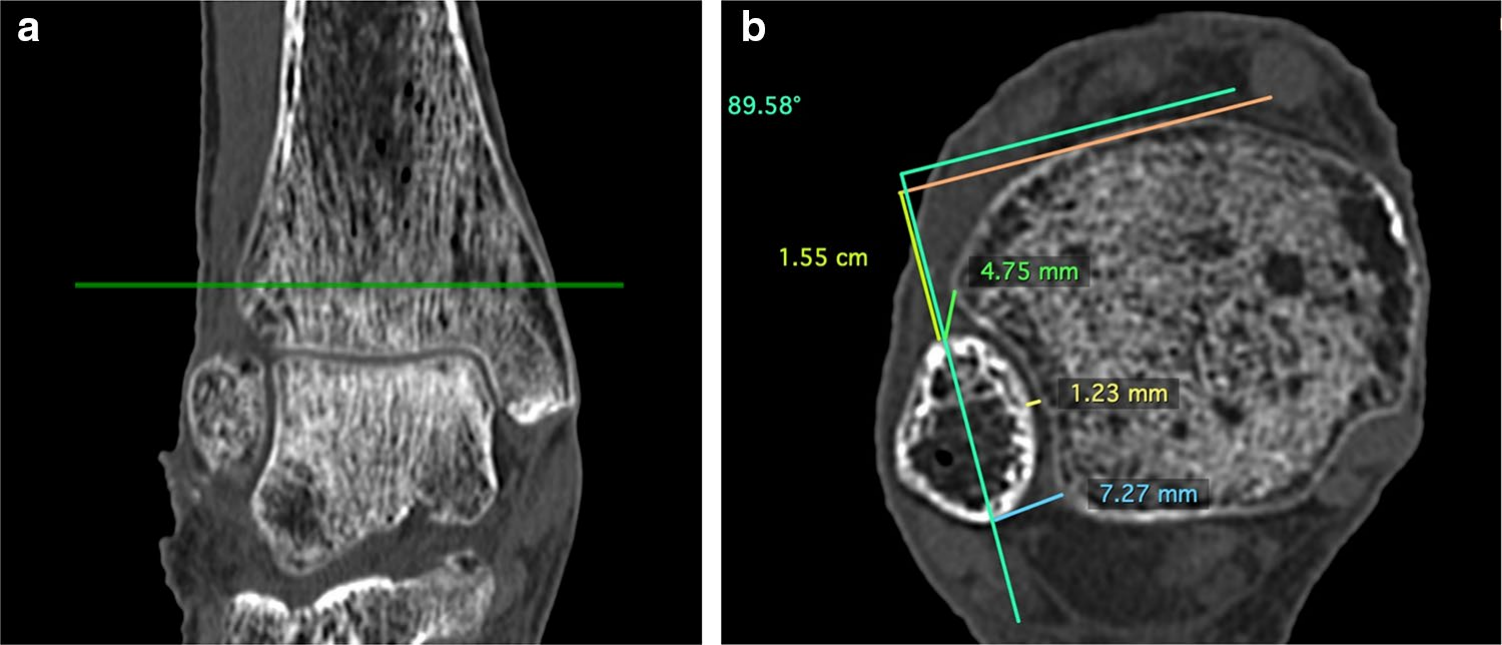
a, b CT scan of an ankle in neutral position. a visualisation of a reference line 1 cm above the joint line in the coronal plane. b  visualisation of the measures: Syn_post (7.27 mm), Syn_trans (1.23 mm), Syn_ant (4.75 mm), Rotation (89,58°) and Translation (1.55 cm). The tangential line just in front of the tibia is taken as a reference line for the measurements of the rotational angle and translation of the fibula [11]
